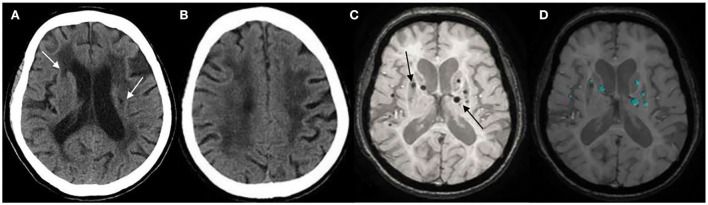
(A) Lacunar infarcts (LIs) are seen in the bilateral basal ganglia on computed tomography (CT) (arrow). (B) Extensive hypodensity in the bilateral corona radiate, and the Blennow score of the LA was 3. (C) susceptibility-weighted imaging (SWI) shows multiple cerebral microbleeds (CMBs; >5) in a 73-years-old female with hypertension for 20 years (arrow). (D) CMBs diagnosed by BioMind AI software on SWI (green marker).
